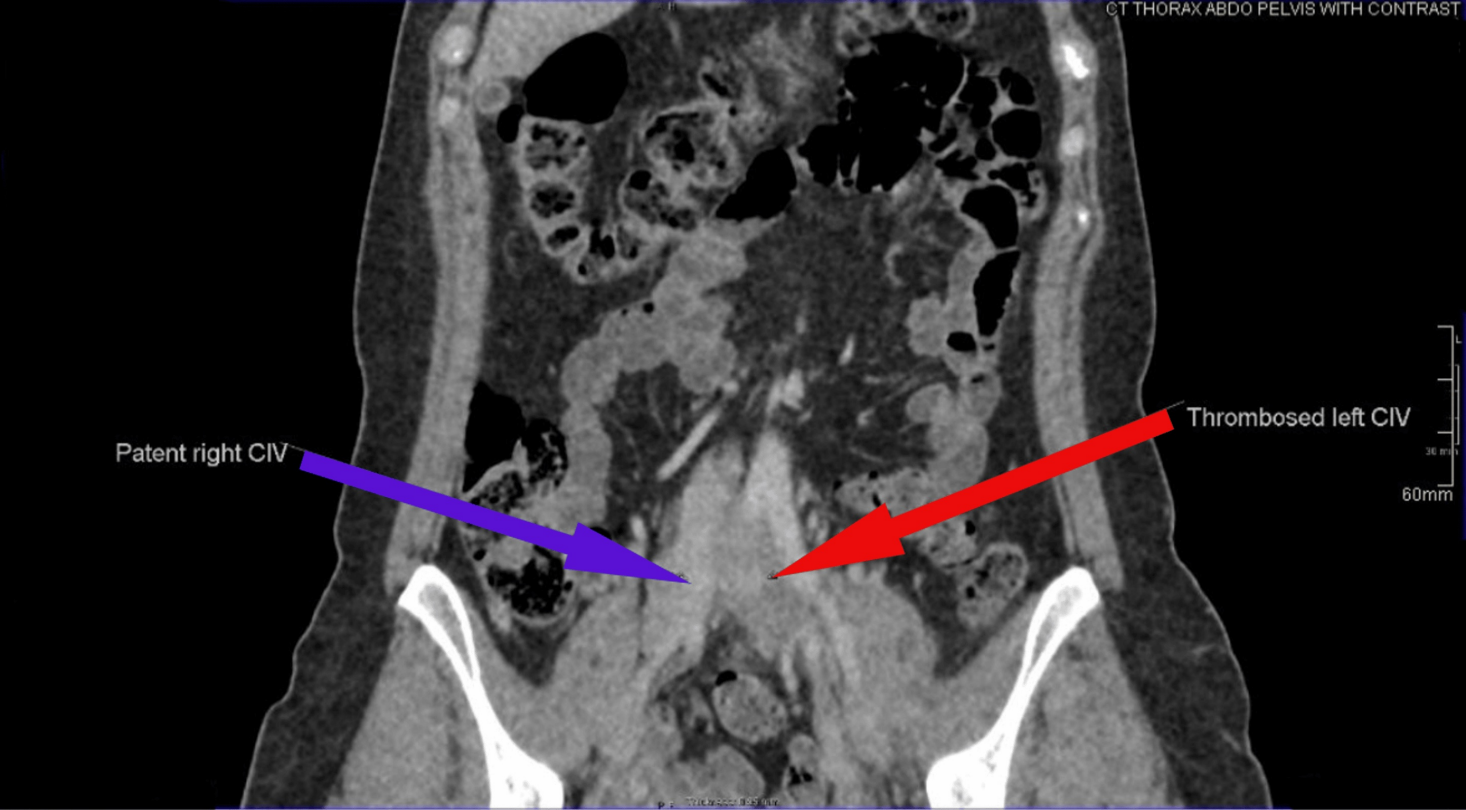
Thrombosed left common iliac vein (CIV) and adjacent patent right CIV seen on CT thorax, abdomen, and pelvis (CT TAP) scan CT: computed tomography
